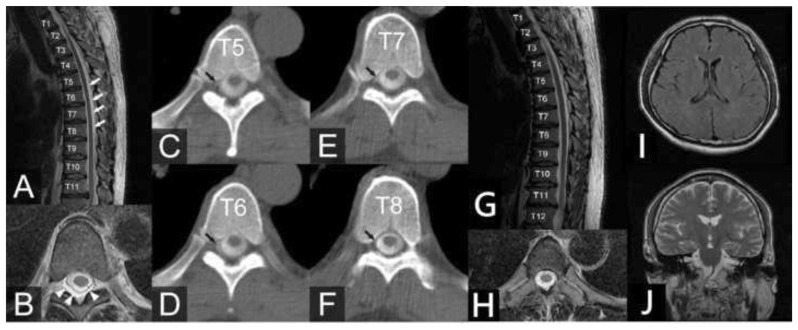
(A) MR sagittal image shows the dura (white arrows) located between the CSF at the T1 to T12 levels. (B) MR axial view shows epidural CSF collection (white arrow heads). (C, D, E, F) Thoracic CT myelography shows contrast leakage at the T5 to T8 levels on the right side (black arrows). (G) The thoracic spine sagittal T2weighted image 6 months after treatment shows no more epidural fluid collection. (H) The thoracic spine MRI axial image 6 months after treatment shows no more epidural fluid collection. (I, J) The brain MRI image 6 months after treatment shows no more subdural fluid collection.
